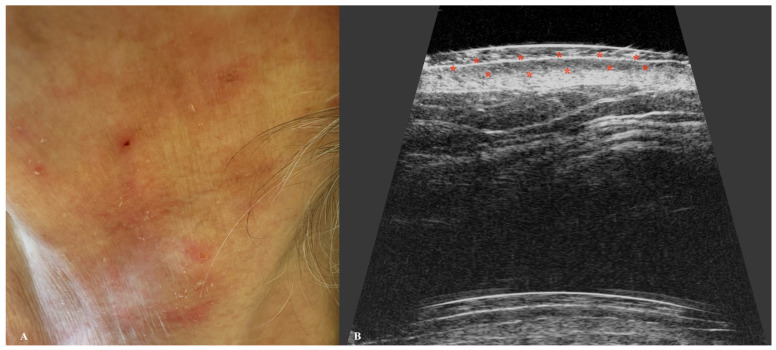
Macroscopic view of early stage mycosis fungicides (A) with a corresponding ultrasonographic image (B). Stars indicate tumor margins.
